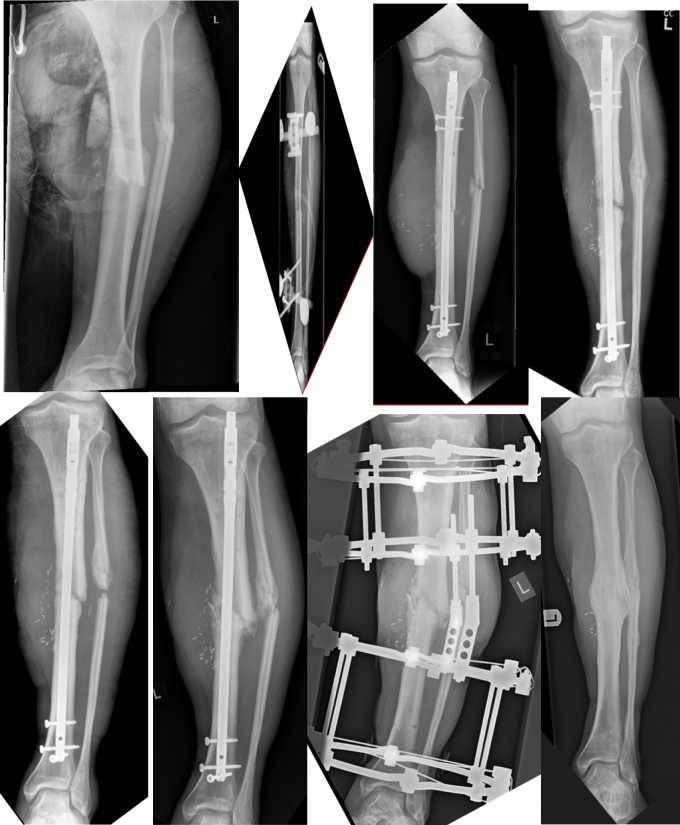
Top left to right: initial injury, temporary external fixator, definitive IMN, subsequent non-union. Bottom left to right: IMN dynamisation, progressive deformity, application of Ilizarov frame and fibular osteotomy, final plain films
